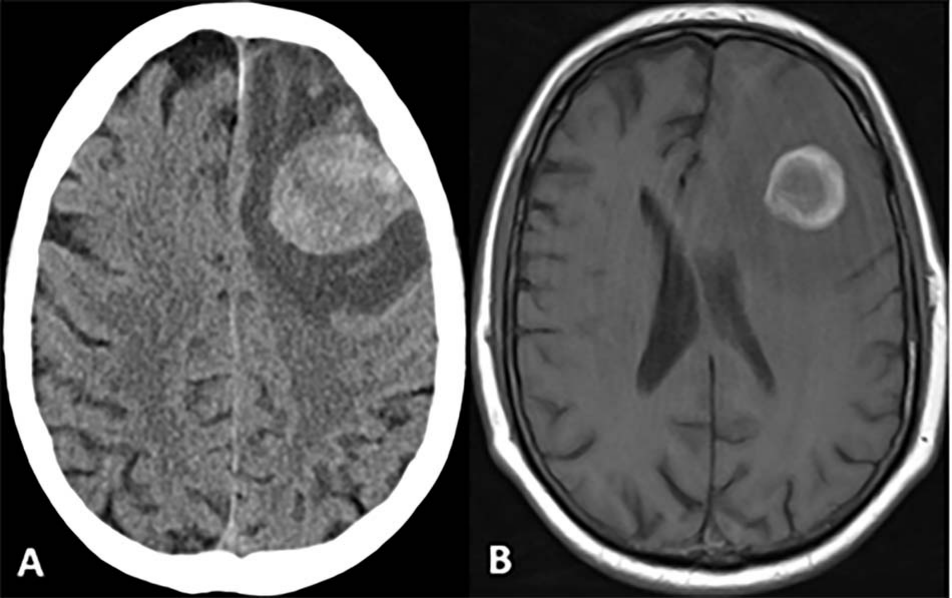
CT and MRI scans of the head indicating a large hemorrhagic enhancing lesion in the left frontal lobe with surrounding edema causing a rightward midline shift.
